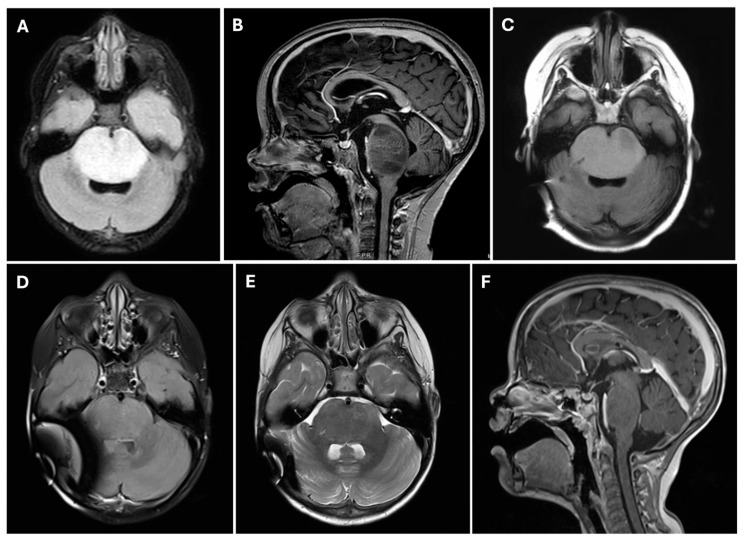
A 4-year-old girl presented with dysconjugate gaze and gain instability. MRI brain images demonstrated an expansile mass of the pons: diffuse intrinsic pontine glioma (DIPG). (A) Axial FLAIR MRI of patient with DIPG demonstrating diffuse hyperintensity involving the brainstem pons. (B) T1 Sagittal MRI with contrast of a patient with DIPG demonstrating expansile hypointensity of the brainstem pons. (C) Stereotactic needle biopsy track along right side of brainstem pons from a transcerebellar approach for DIPG biopsy. (D–F) Treatment effects of chimeric antigen receptor (CAR) T-cells on diffuse intrinsic pontine glioma (DIPG) at 3 months after the administration of CAR T-cell therapy. (D) Axial FLAIR image and (E) axial T2 image demonstrating significant reduction in hyperintense signal in the pons and marked reduction in tumor size compared to pretreatment images in Image A. (F) Sagittal T1 image with contrast administration demonstrating marked decrease in expansile pontine mass compared to pretreatment images in Image B.
